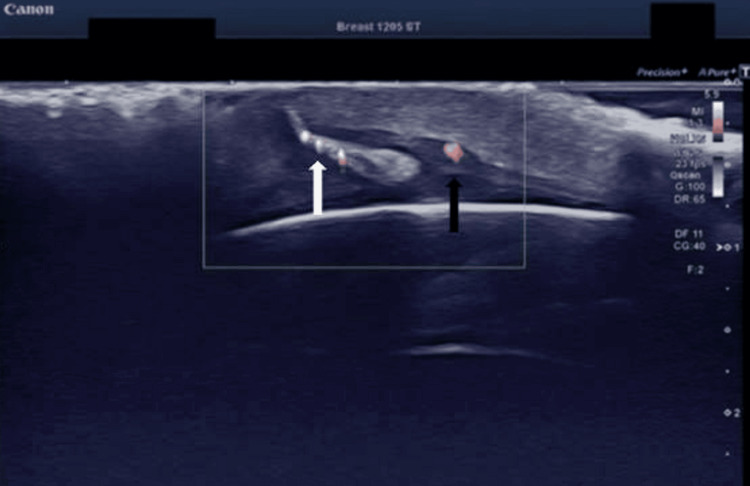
Color Doppler image reveals the vascularity of the larval structure (white arrow) and the accompanying hyperemia of the subcutaneous tissue (black arrow) nearby
